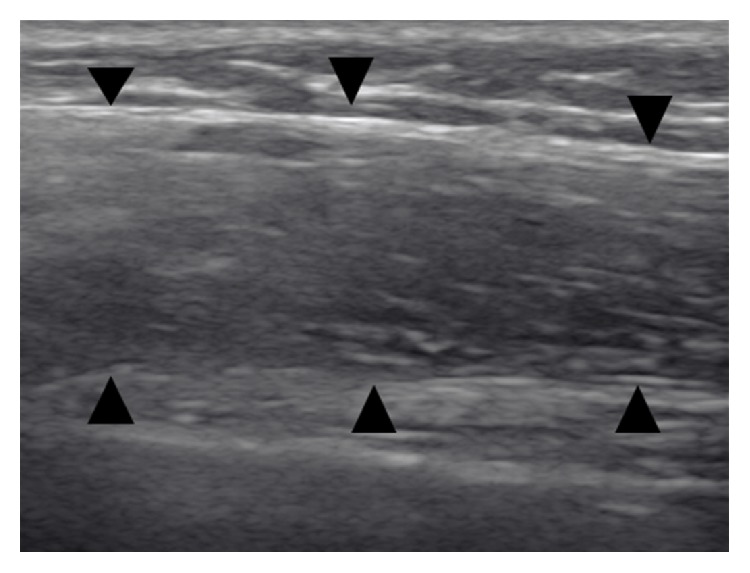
Case  2. Thoracic ultrasonography at the level of the middle third of left thorax shows a thick hypoechoic band with fine echoes, reflecting thickening of the visceral pleura (arrowheads).
